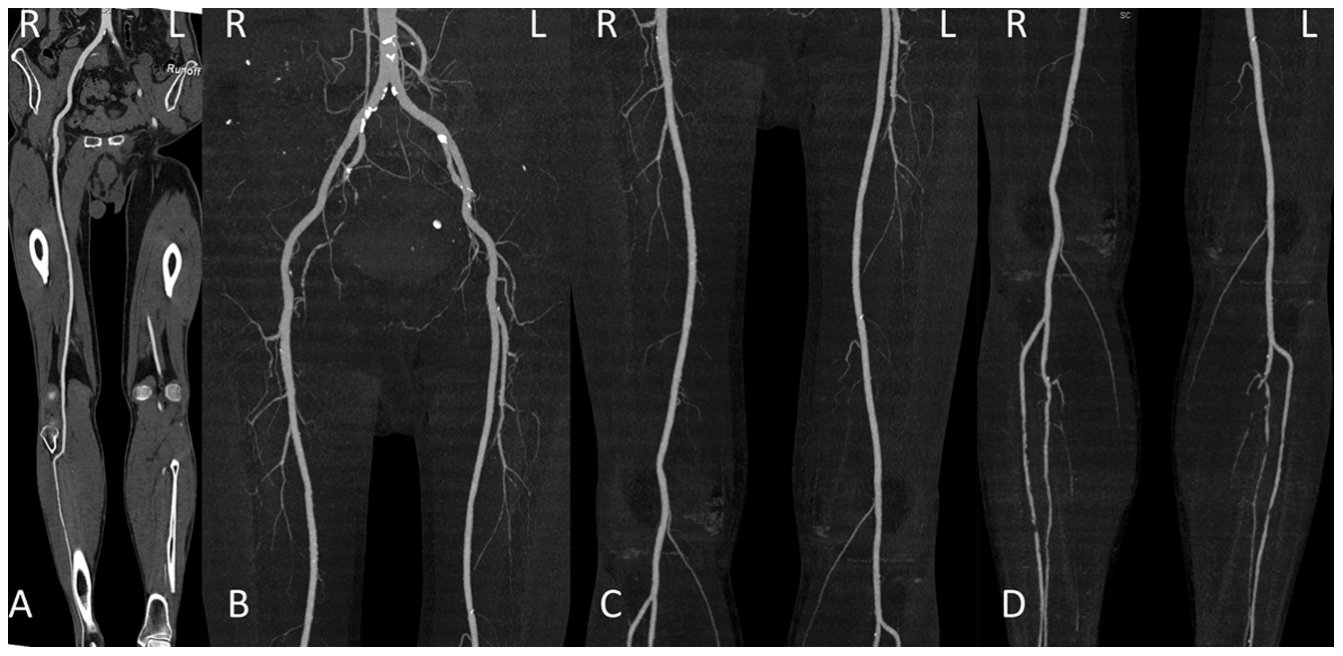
Lower extremity CTA using 120-year-old man with PAD. A, curved planar reformatted image and B–D, maximum intensity projection images. The image quality was visually classified as score 4 (excellent) in the aortoiliac (B), femoropopliteal (C) and lower leg segments (D).
